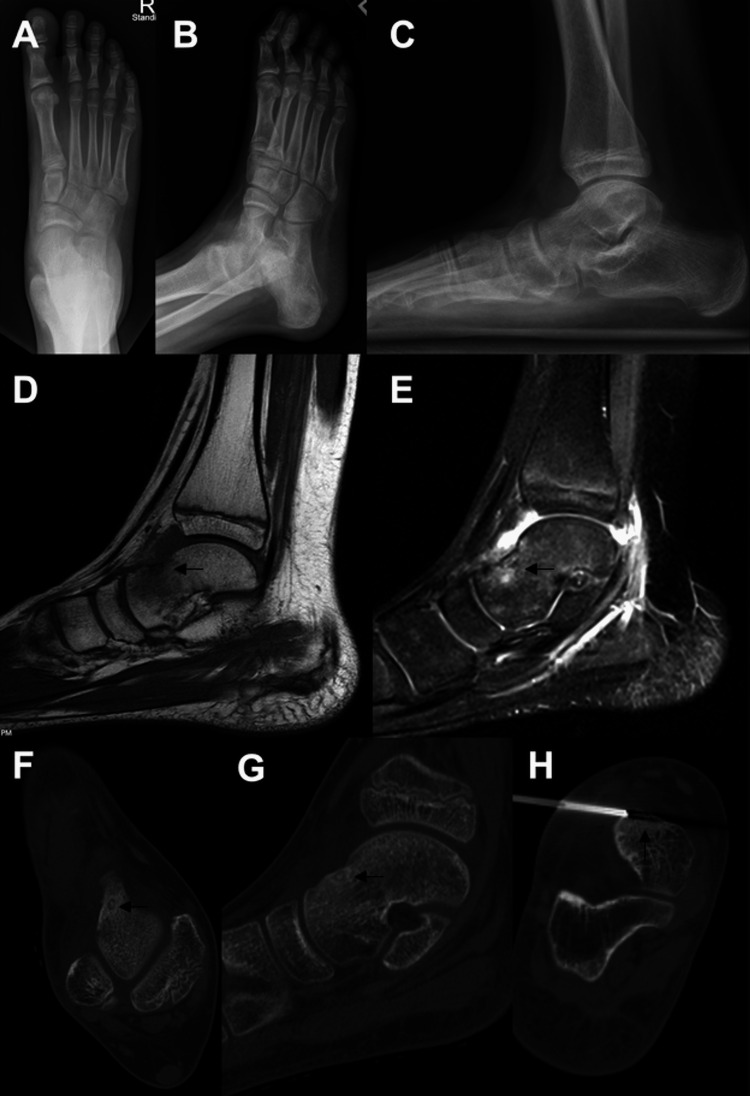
Images of the patient discussed in case four. Plain radiographs (AP (A), oblique (B), and lateral (C)) of the foot demonstrated no abnormality. Sagittal T1 MRI demonstrated a low signal area (D) with extensive edema on the fluid-sensitive sequence (E). CT confirmed the lesion with classic characteristics on axial (F) and sagittal (G) images. The patient was treated definitively with RFA (H) of the lesion under CT guidance. MRI: magnetic resonance imaging; CT: computed tomography; RFA: radio-frequency ablation
